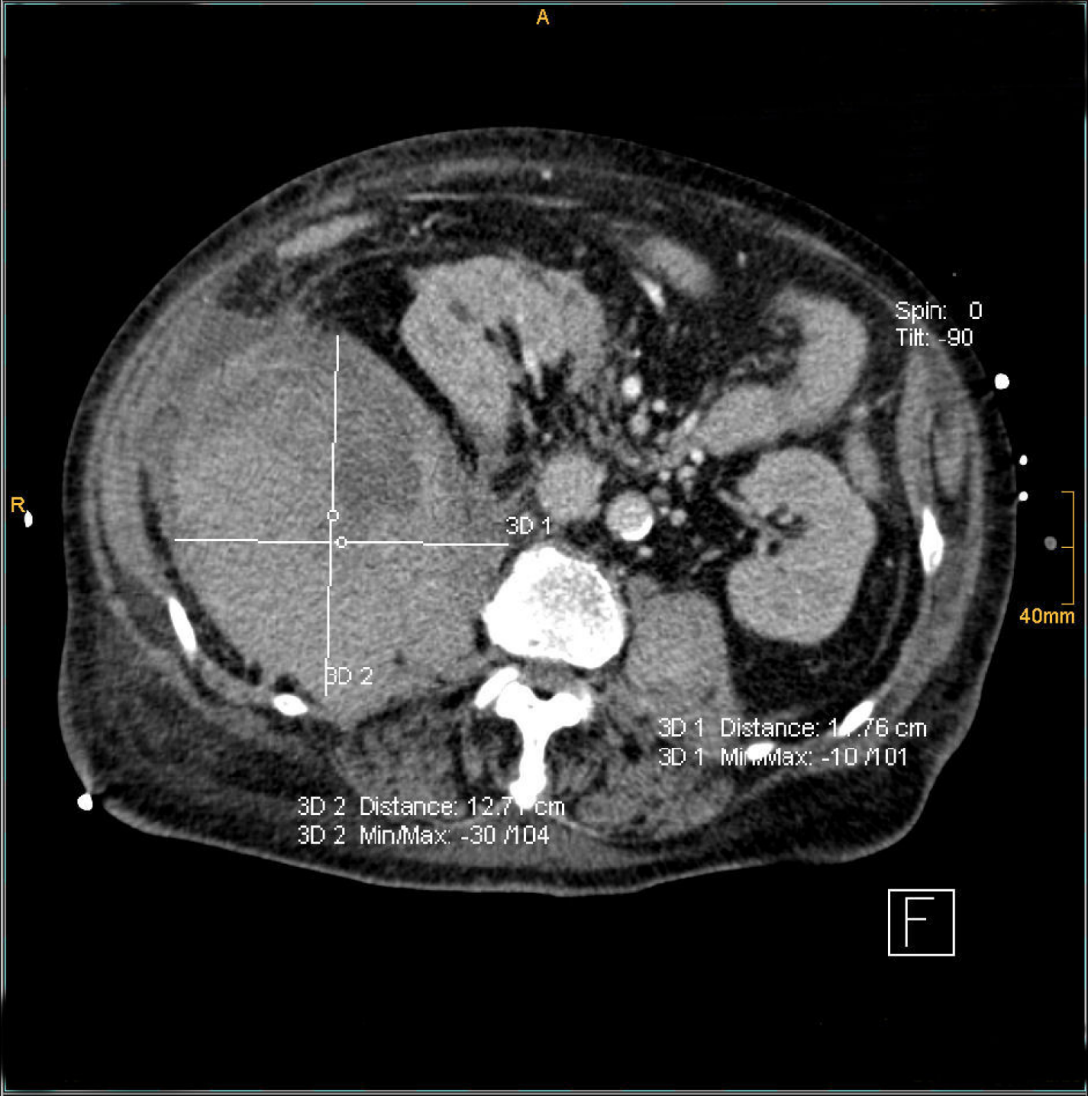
3^rd ^case. CT - axial plan of the hematoma.
